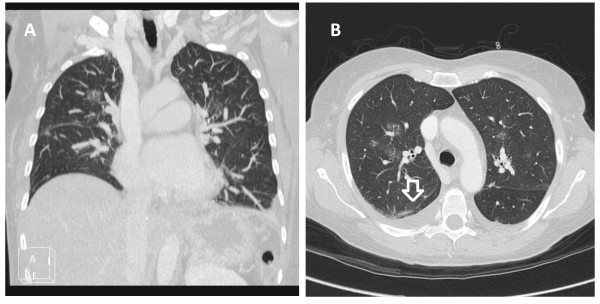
Chest CT scan showing evidence of pulmonary edema (A-B).
